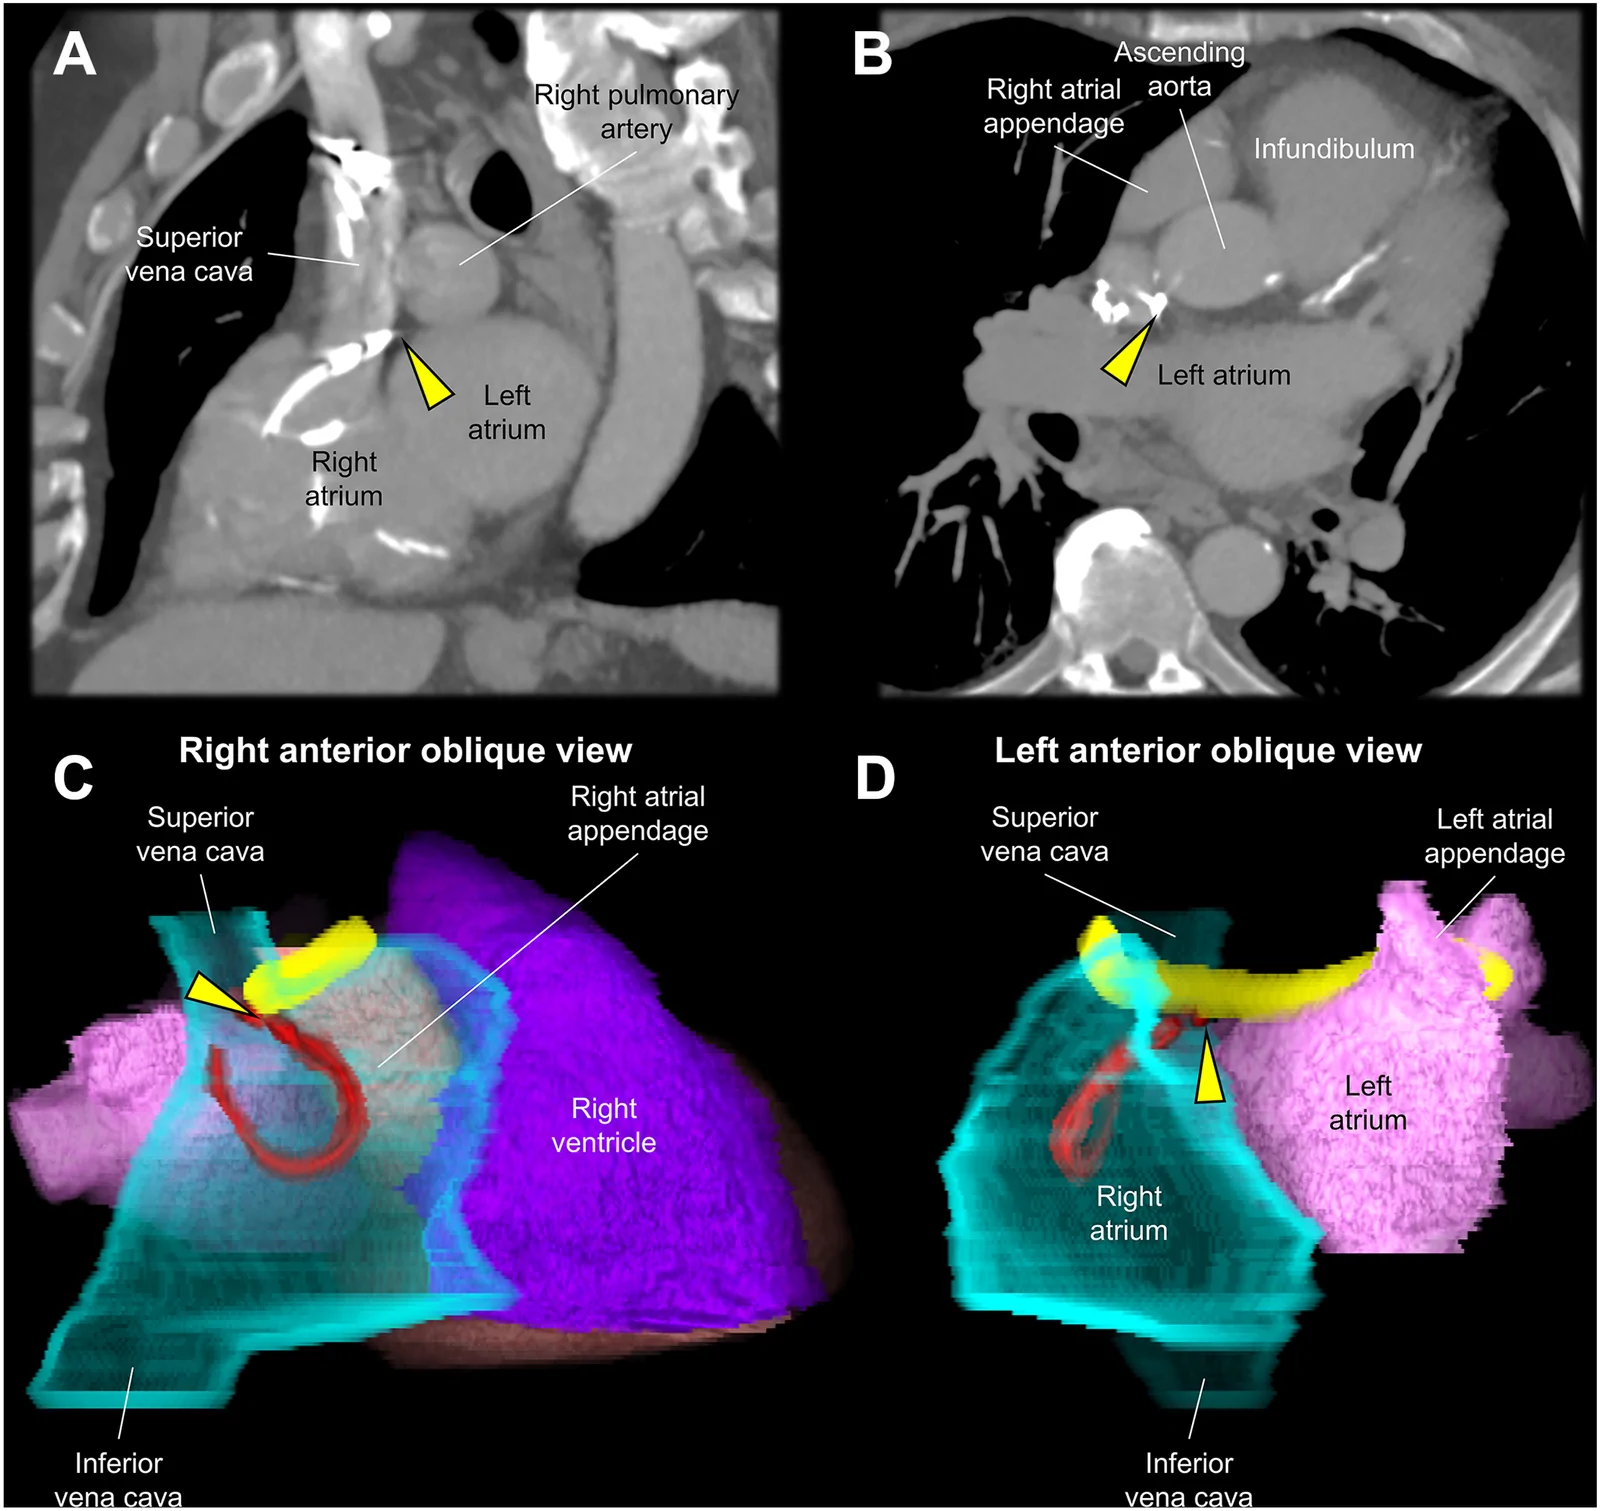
Contrast-enhanced thoracic computed tomography after Bachmann’s bundle pacing lead implantation. Multiplanar reconstruction images viewed from the inferior (A) and left anterior oblique (B) views depict the tip of the lead placed at the posteromedial area of the atriocaval junction adjacent to the interatrial portion of Bachmann’s bundle covering the anterosuperior interatrial groove. Volume-rendered images viewed from the right (C) and left (D) anterior oblique directions, reconstructed with virtual transverse sinus (*yellow*) and Bachmann’s pacing lead (*red*), depict the relationship between the lead and surrounding structures relative to the transverse sinus.
